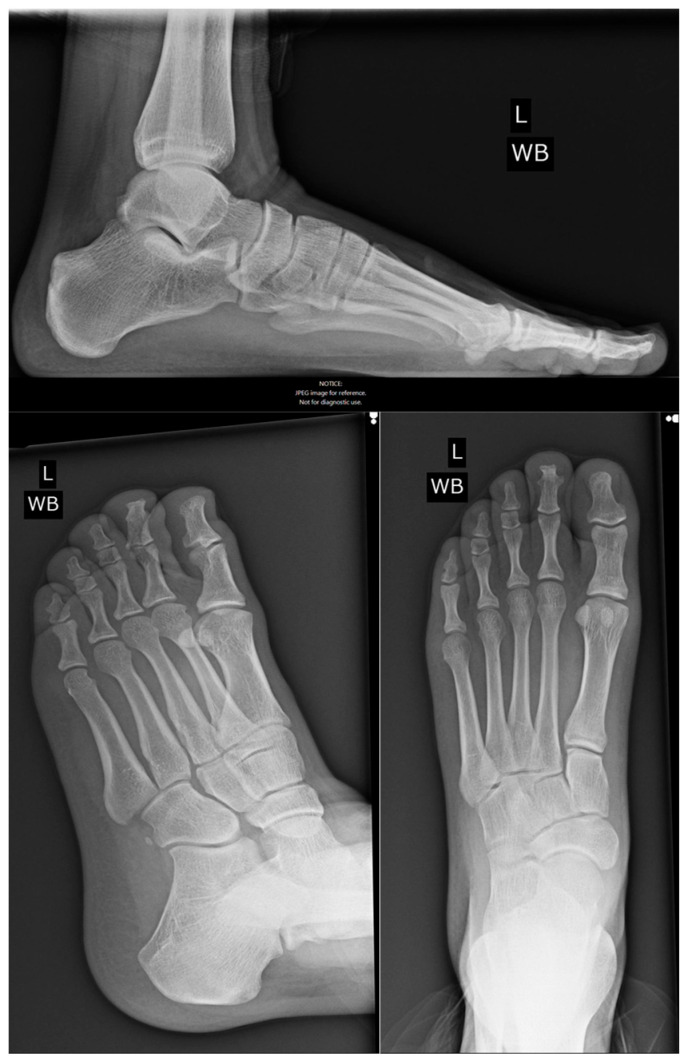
One-year postoperative radiograph showing maintained fusion and restored toe profile.
